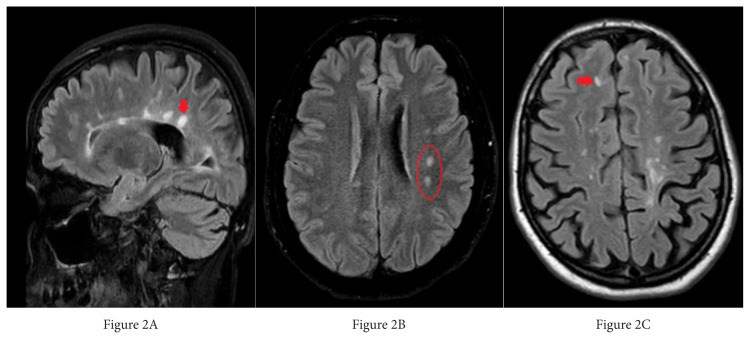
White matter lesion locations: A) Sagittal 3D-FLAIR sequence, periventricular lesion, B) Axial 2D-FLAIR sequence, deep white matter lesion, C) Axial 2D-FLAIR sequence, juxtacortical lesion.
